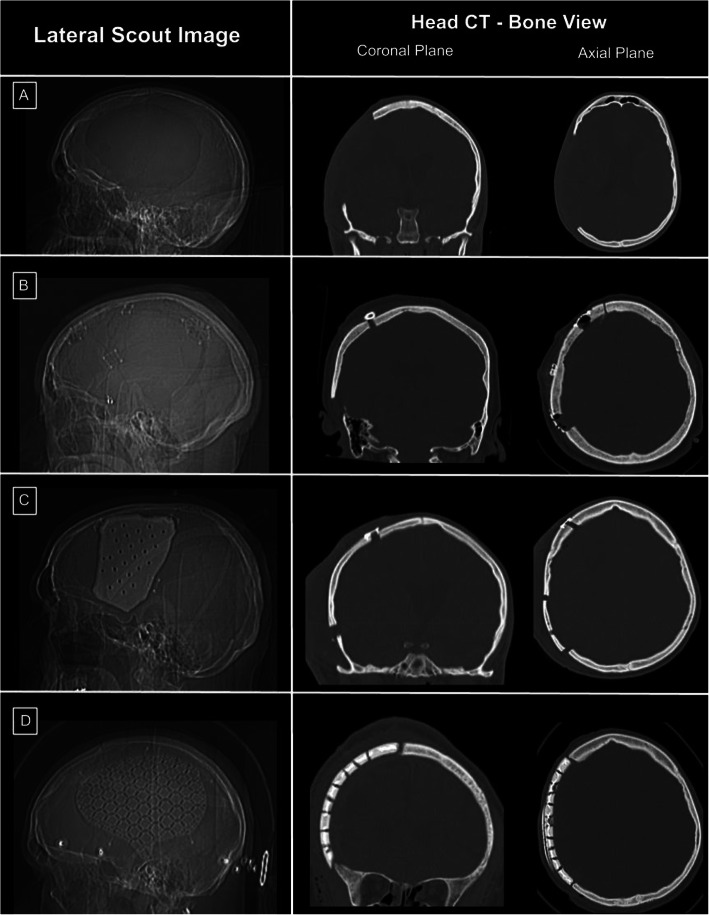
Head CT Images of Autologous, Synthetic, and 3D Printed Cranioplasty Implants (a) Images of a subject post-craniectomy. (b) Images of a subject post-cranioplasty with an autologous implant. Note the defect at the inferior border of the implant in the coronal plane. (c) Images of a subject post-cranioplasty with a synthetic implant. Note the improved profile of the skull border using the synthetic implant in the coronal plane as well as the remaining inferior defect. (d) Images of a subject post-cranioplasty with a 3D printed implant. Note the improved defect repair and restoration of the native skull border profile
